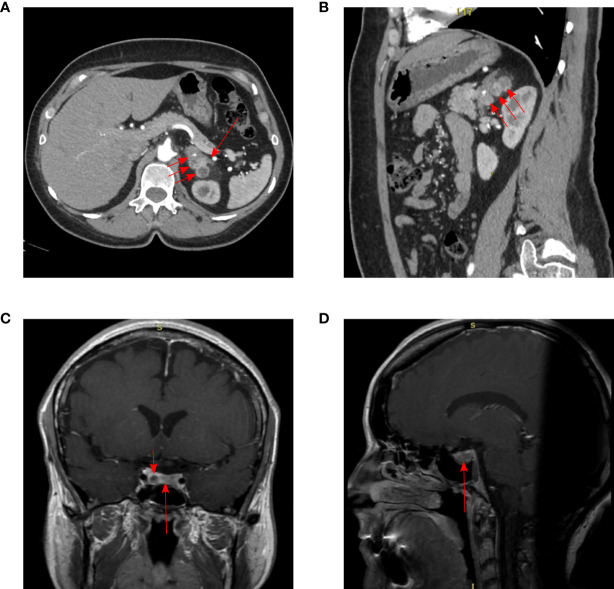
Imaging results in our patient. CT of the abdomen: (A) arterial phase, axial projection, (B) arterial phase, sagittal projection. Three pheochromocytomas in the body and medial pedicle of the left adrenal gland (short arrows). Uniformly thickened lateral pedicle of the left adrenal gland (long arrow). The adrenal lesions were located close to each other, 21 × 22 × 23 mm, 24 × 14 × 19 mm and 24 × 22 × 24 mm. MRI of pituitary adenoma (long arrow) with cystic component (short arrow), subtotally replaces adenohypophysis: (C) T1 CE (contrast-enhanced) coronal projection, (D) T1 CE sagittal projection. The size of pituitary adenoma was 22 × 8 × 14.8 mm.
